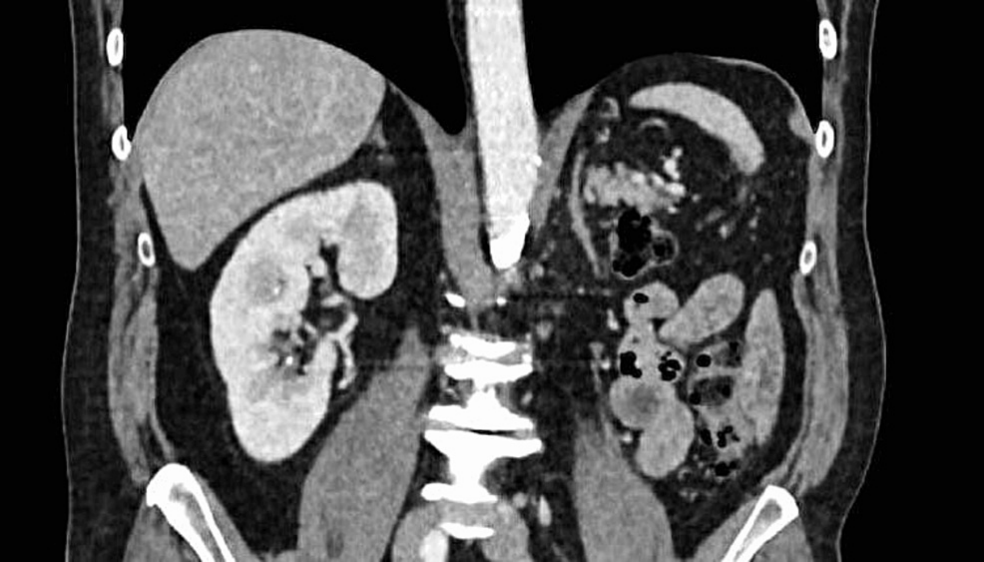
Coronal CT scan showing a well-healed left nephrectomy bed with no evidence of recurrent abscess.
